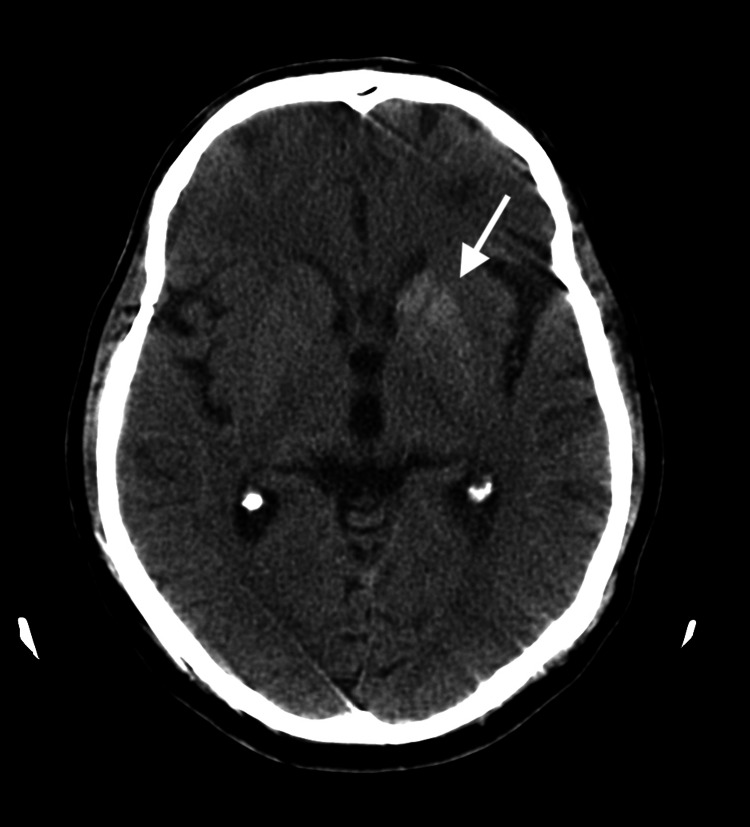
Head CT scan of case 2 showing a hyperdensity of the left globus pallidus.
